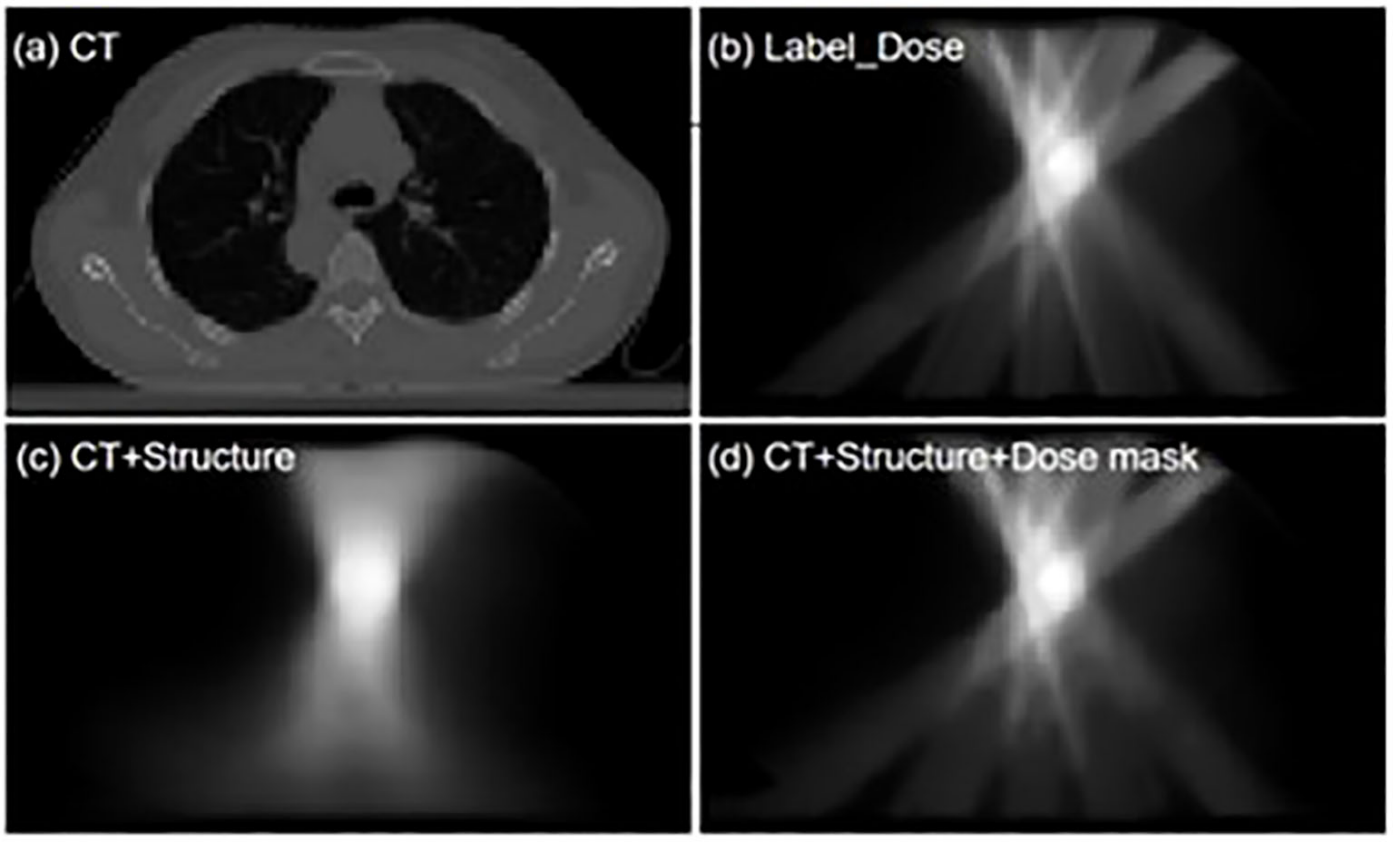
'Visualization comparison of dose distribution predictions between the conventional input model and the dose mask-assisted model. (a) CT image of a sample patient; (b) Dose distribution of the clinical treatment plan; (c) Model-predicted dose distribution with CT and anatomical structures as sole inputs; (d) Model-predicted dose distribution with additional dose mask input.
